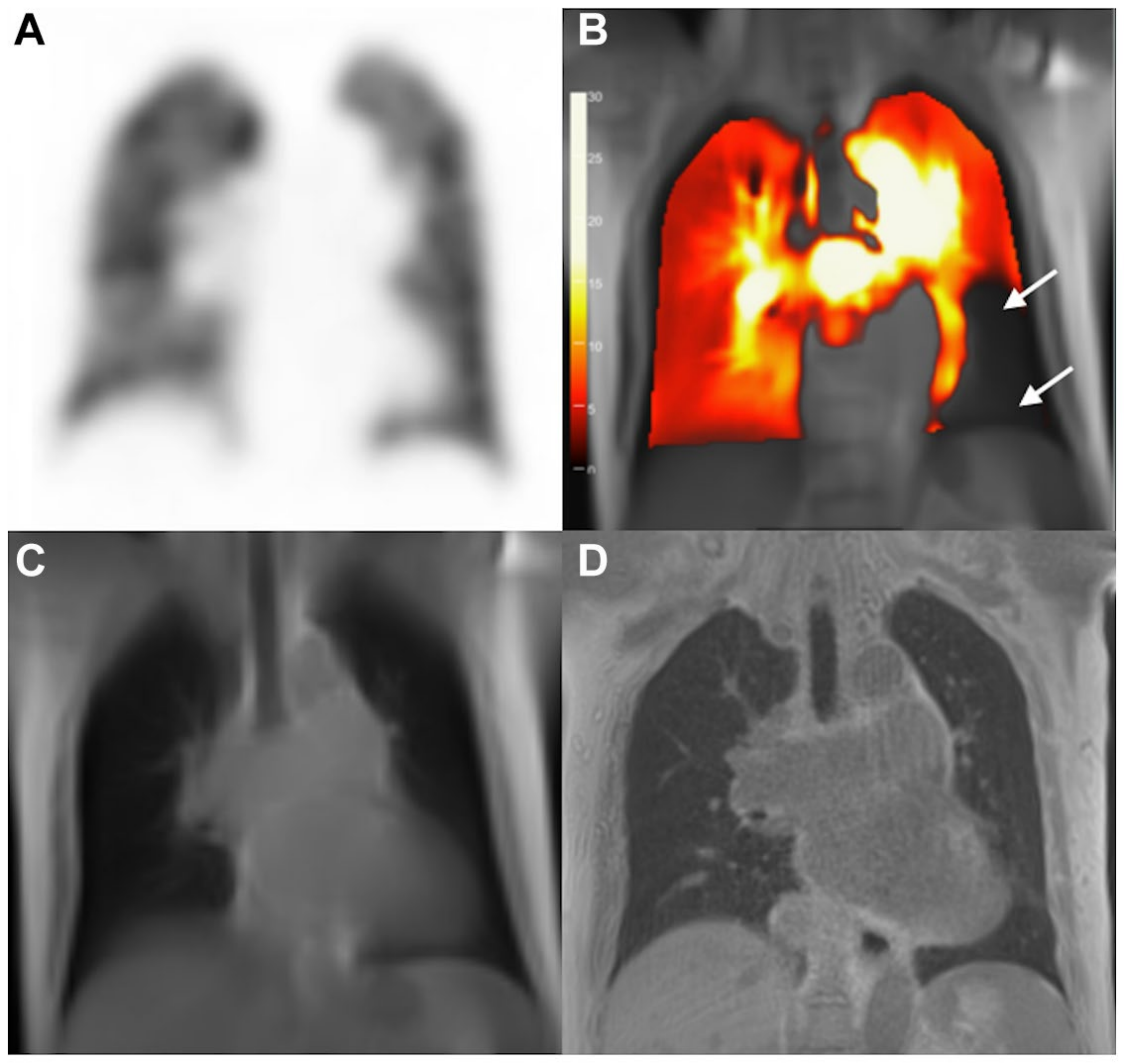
Images of a 75-year-old woman with pulmonary hypertension. SPECT perfusion image (A) reveals normal perfusion and corresponding PW-PREFUL MRI (B) reveals a false-positive diagnosis for PE. Arrow-marked areas indicate a perfusion defect in the left segment V and segment VII/VIII. (C) bSSFP, and (D) 3D-UTE; bSSFP, Balanced steady-state free precession; 3D-UTE, 3-dimensional ultrashort echo time; MRI, magnetic resonance imaging; PE, pulmonary embolism; PREFUL, phase-resolved functional lung; PW, perfusion-weighted; SPECT, single-photon emission computed tomography.
